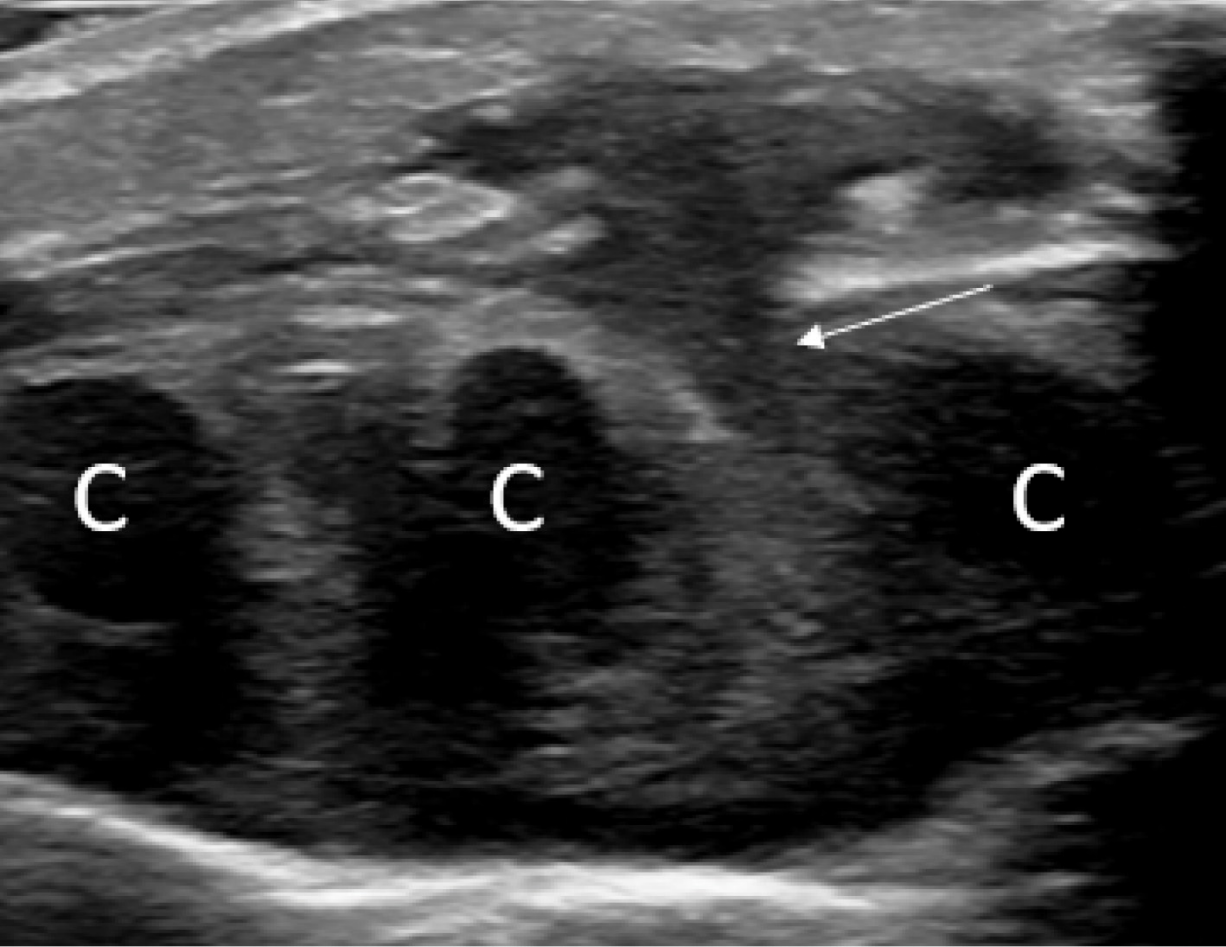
Ultrasound confirmed a soft tissue lesion arising from the space between the fifth and sixth intercostal cartilage, referred to as the ‘dumbbell sign’.
